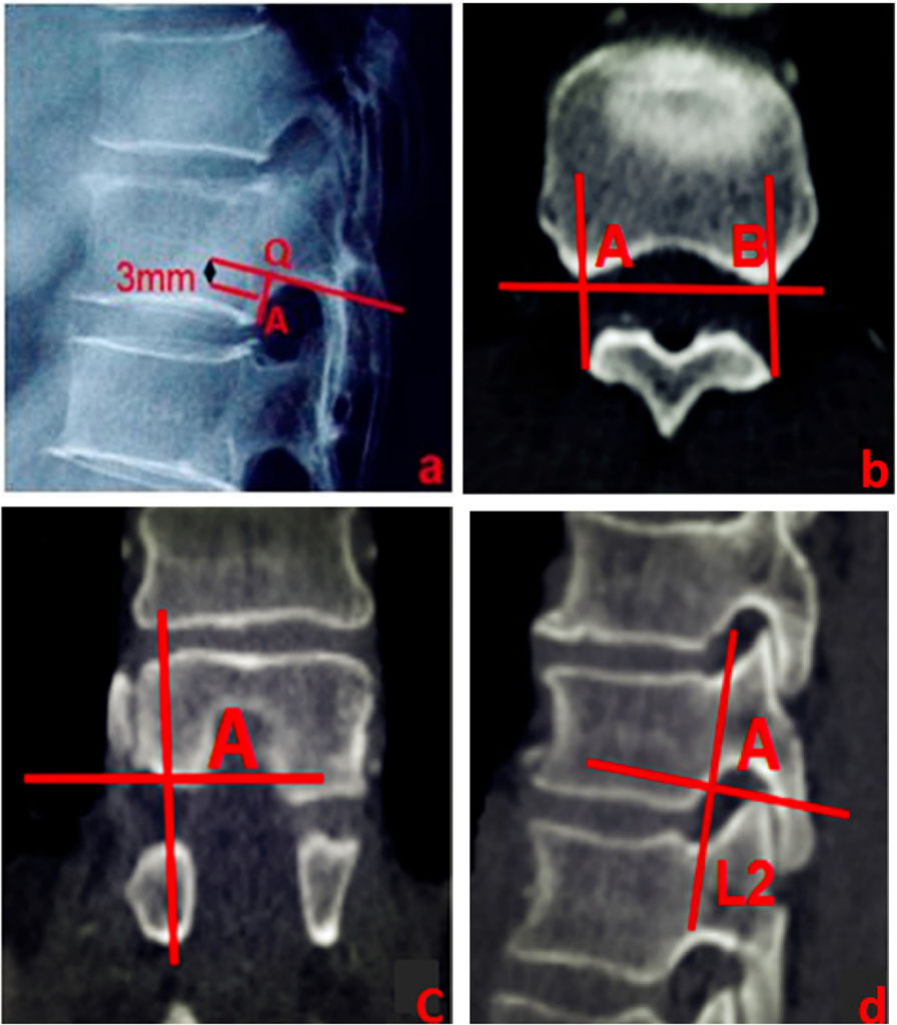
a Cross inferior vertebral notch to make the corresponding parallel line of the inferior endplate of the vertebral body and intersect the vertebral body at point Q. Point A, 3 mm below point Q, was selected as the observation point on the posterior vertebral body margin to determine whether bone cement entered the spinal canal. b Posterior margin bone cortex points A and B of the thoracic vertebral body were determined on the axial CT view at the same level as in a. c Point A was reconfirmed on the coronal view by a synchronous localization technique. d Line L2 at point A is perpendicular to the inferior endplate on the sagittal view
